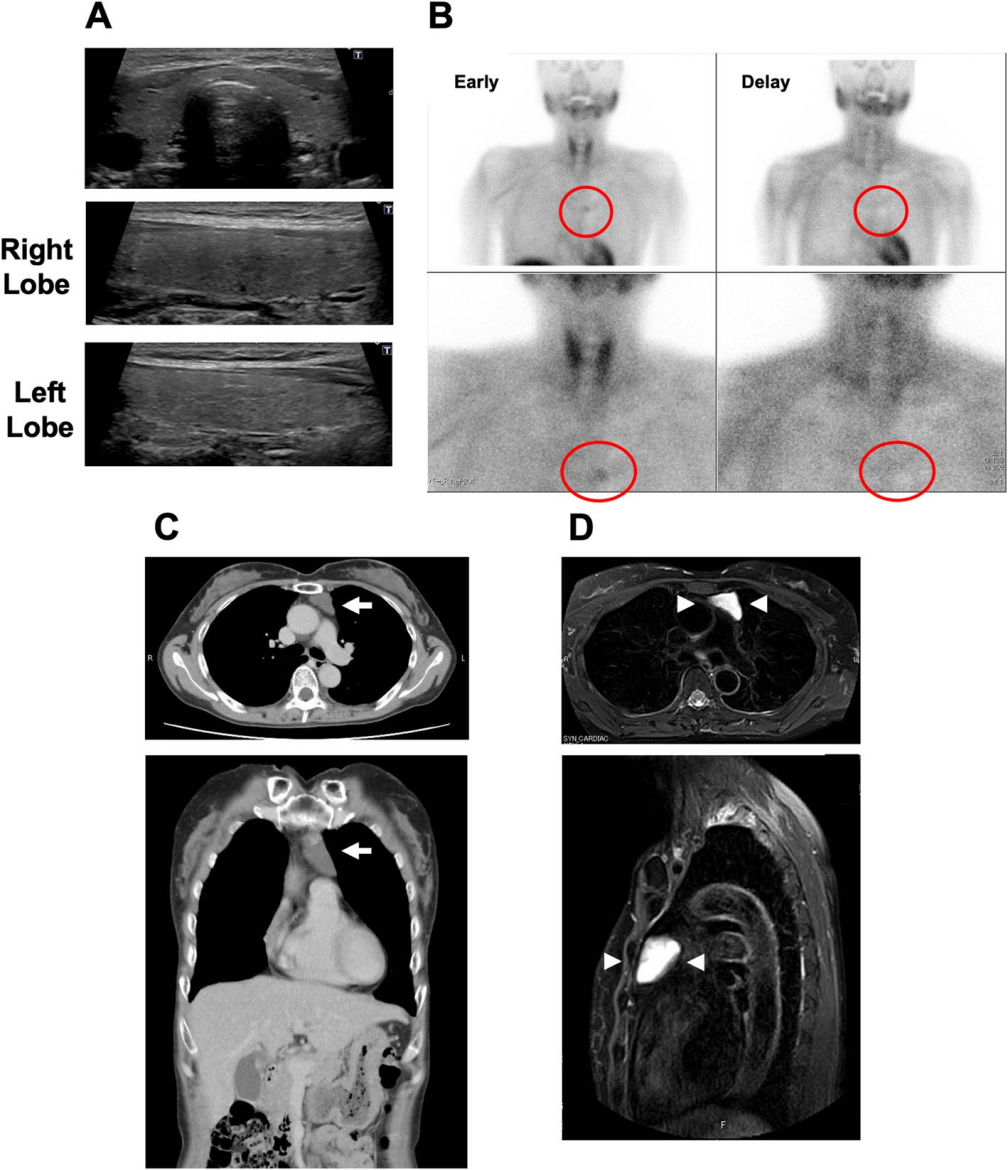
Local diagnosis of ectopic parathyroid cyst inside the thymus in ultrasound examination. (A) ^99m^Tc-methoxy-isobutyl-isonitrile scintigraphy (99mTc-MIBI) (B), enhanced computed tomography (CT) (C) and magnetic resonance imaging (MRI) (D). 99mTc-MIBI confirmed a localized and slightly hyperfunctioning parathyroid tissue in the anterior mediastinum only in an early phase, but not in a delay phase (red circle). Enhanced CT showed a cyst-like tumor in the upper anterior mediastinum (white arrow). The diameter was as large as 50 mm. MRI showed high signal on T1-weighted images due to fluid cyst contents (white arrow head) as observed in 99mTc-MIBI
